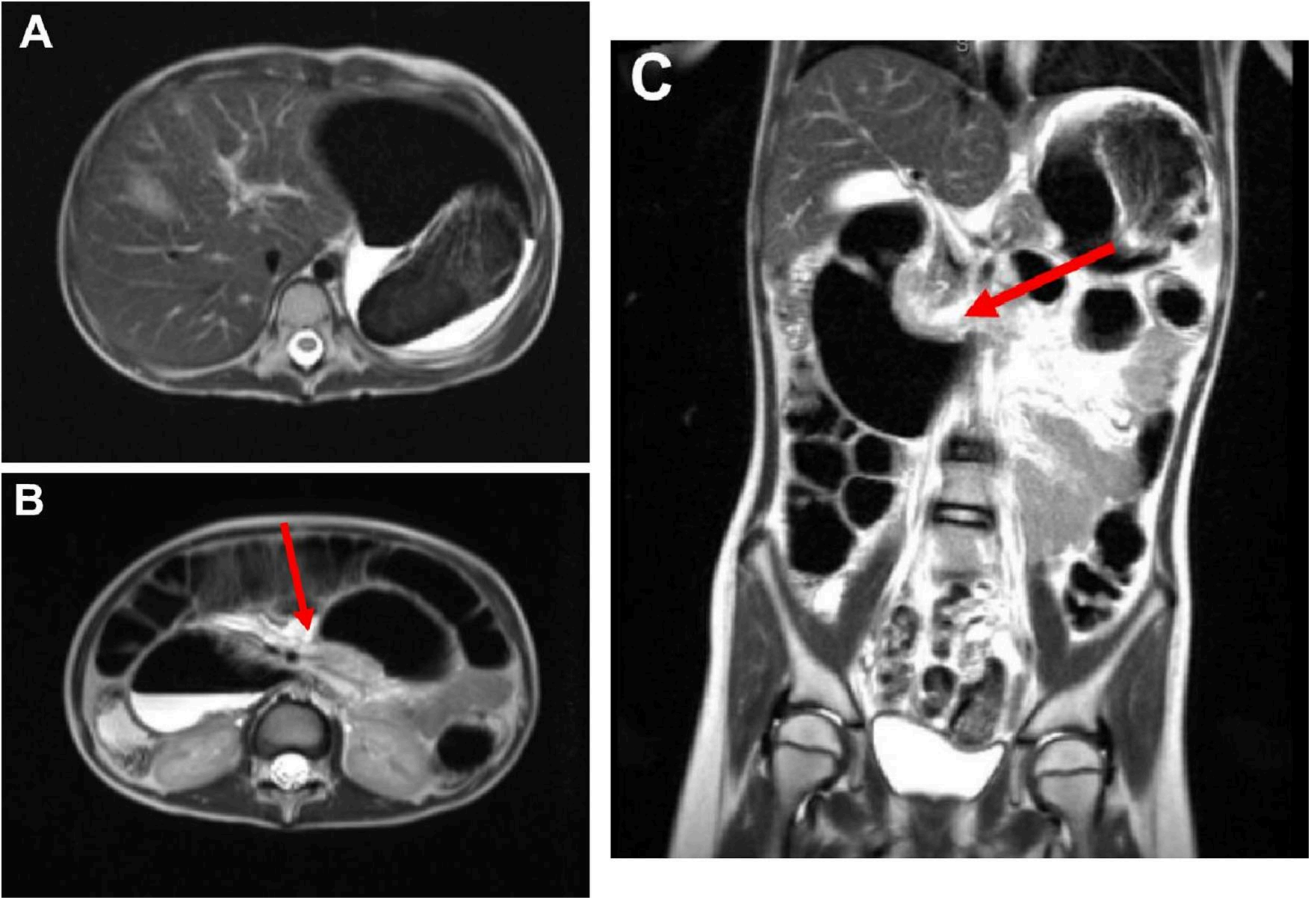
MRI of the abdomen and pelvis. (A) An axial view of a T2 low signal mass-like structure in the gastric fundus. (B) Dilation of the second and proximal third portions of the duodenum with severe narrowing at the level of the SMA. (C) A coronal view showing duodenal dilation with narrowing in the region of the SMA.
